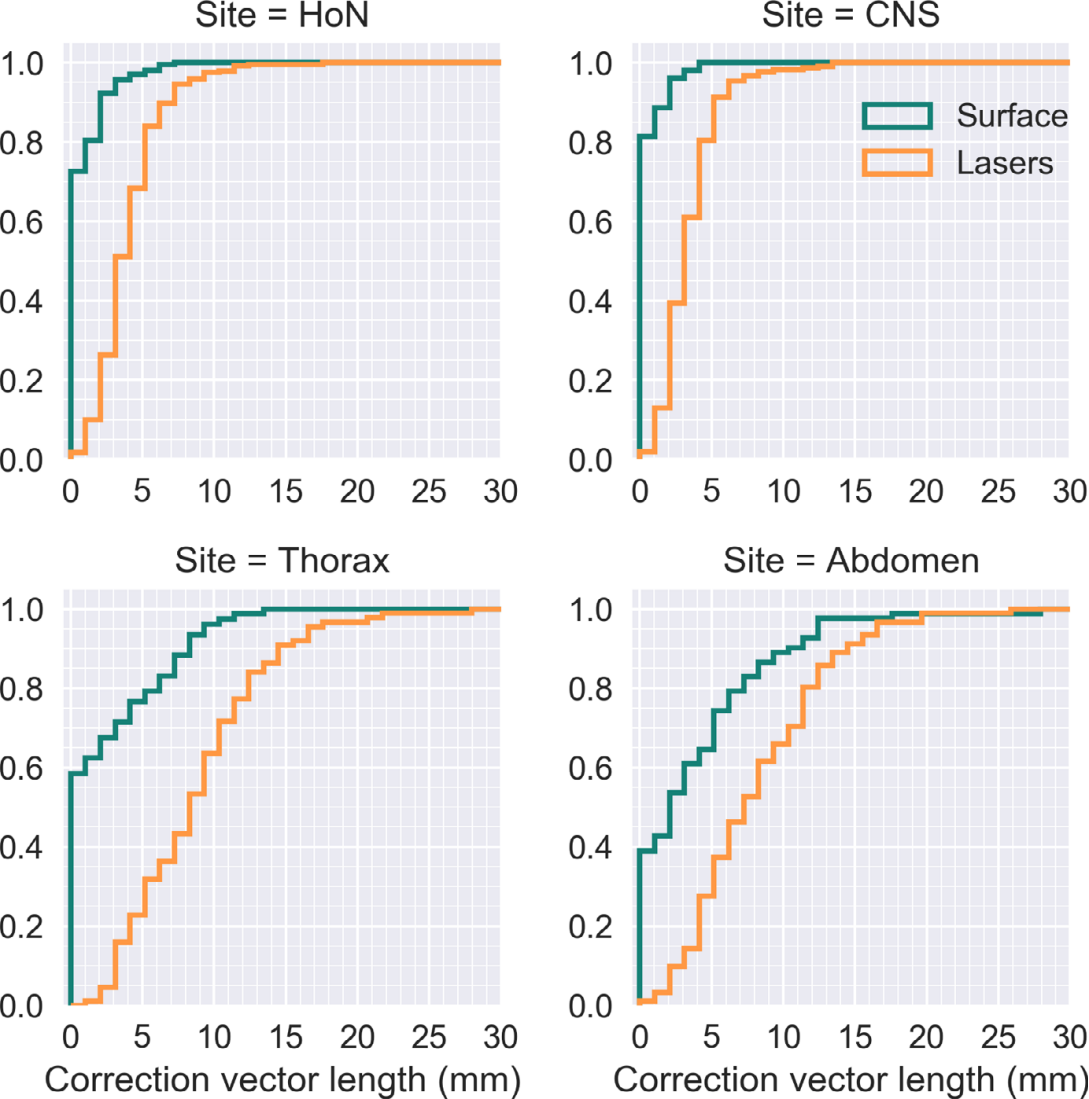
[Length of setup deviation per image modality] residual error for in‐room lasers and surface scanning as assessed by the sum of the megavoltage computed tomography (MVCT) and Sentinel correction vector (orange) and the MVCT setup correction vector (green) respectively. Here plotted as the cumulative sum of the setup correction deviation
